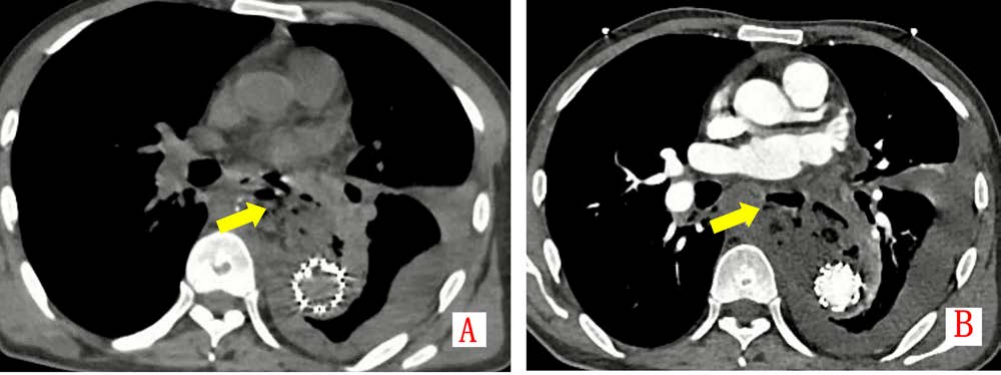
(A, B) Non-contrast CT and CTA exhibit flocculent hyperdensities, gas accumulation around the stent, and a 5.0 cm fistulous tract connecting to the esophagus, consistent with infected aorto-esophageal fistula, alongside moderate left encapsulated hemothorax.
